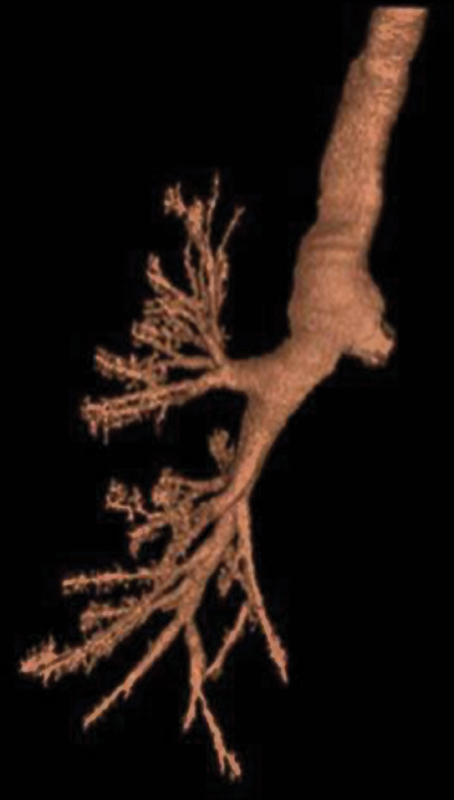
Computed tomography chest airways tree reconstruction showing left-side hypoventilatory phenomenon.
